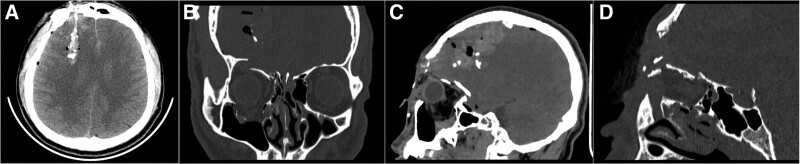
Preoperative CT images. (A) Axial slice showing the location of the skull break, injury tract, and foreign body. (B) Coronal image revealing the fractures of the superior and inferior walls of the orbit, as well as the downward collapse of the eye and its contents. (C) Sagittal image demonstrating air and multiple fragments of bone inside the brain, local hematoma complication, and fractures of the superior orbital wall and anterior skull base. (D) Sagittal reconstructed CT image with the break located in the right frontal sinus, superior orbital wall, and anterior skull base. CT = computed tomography.
